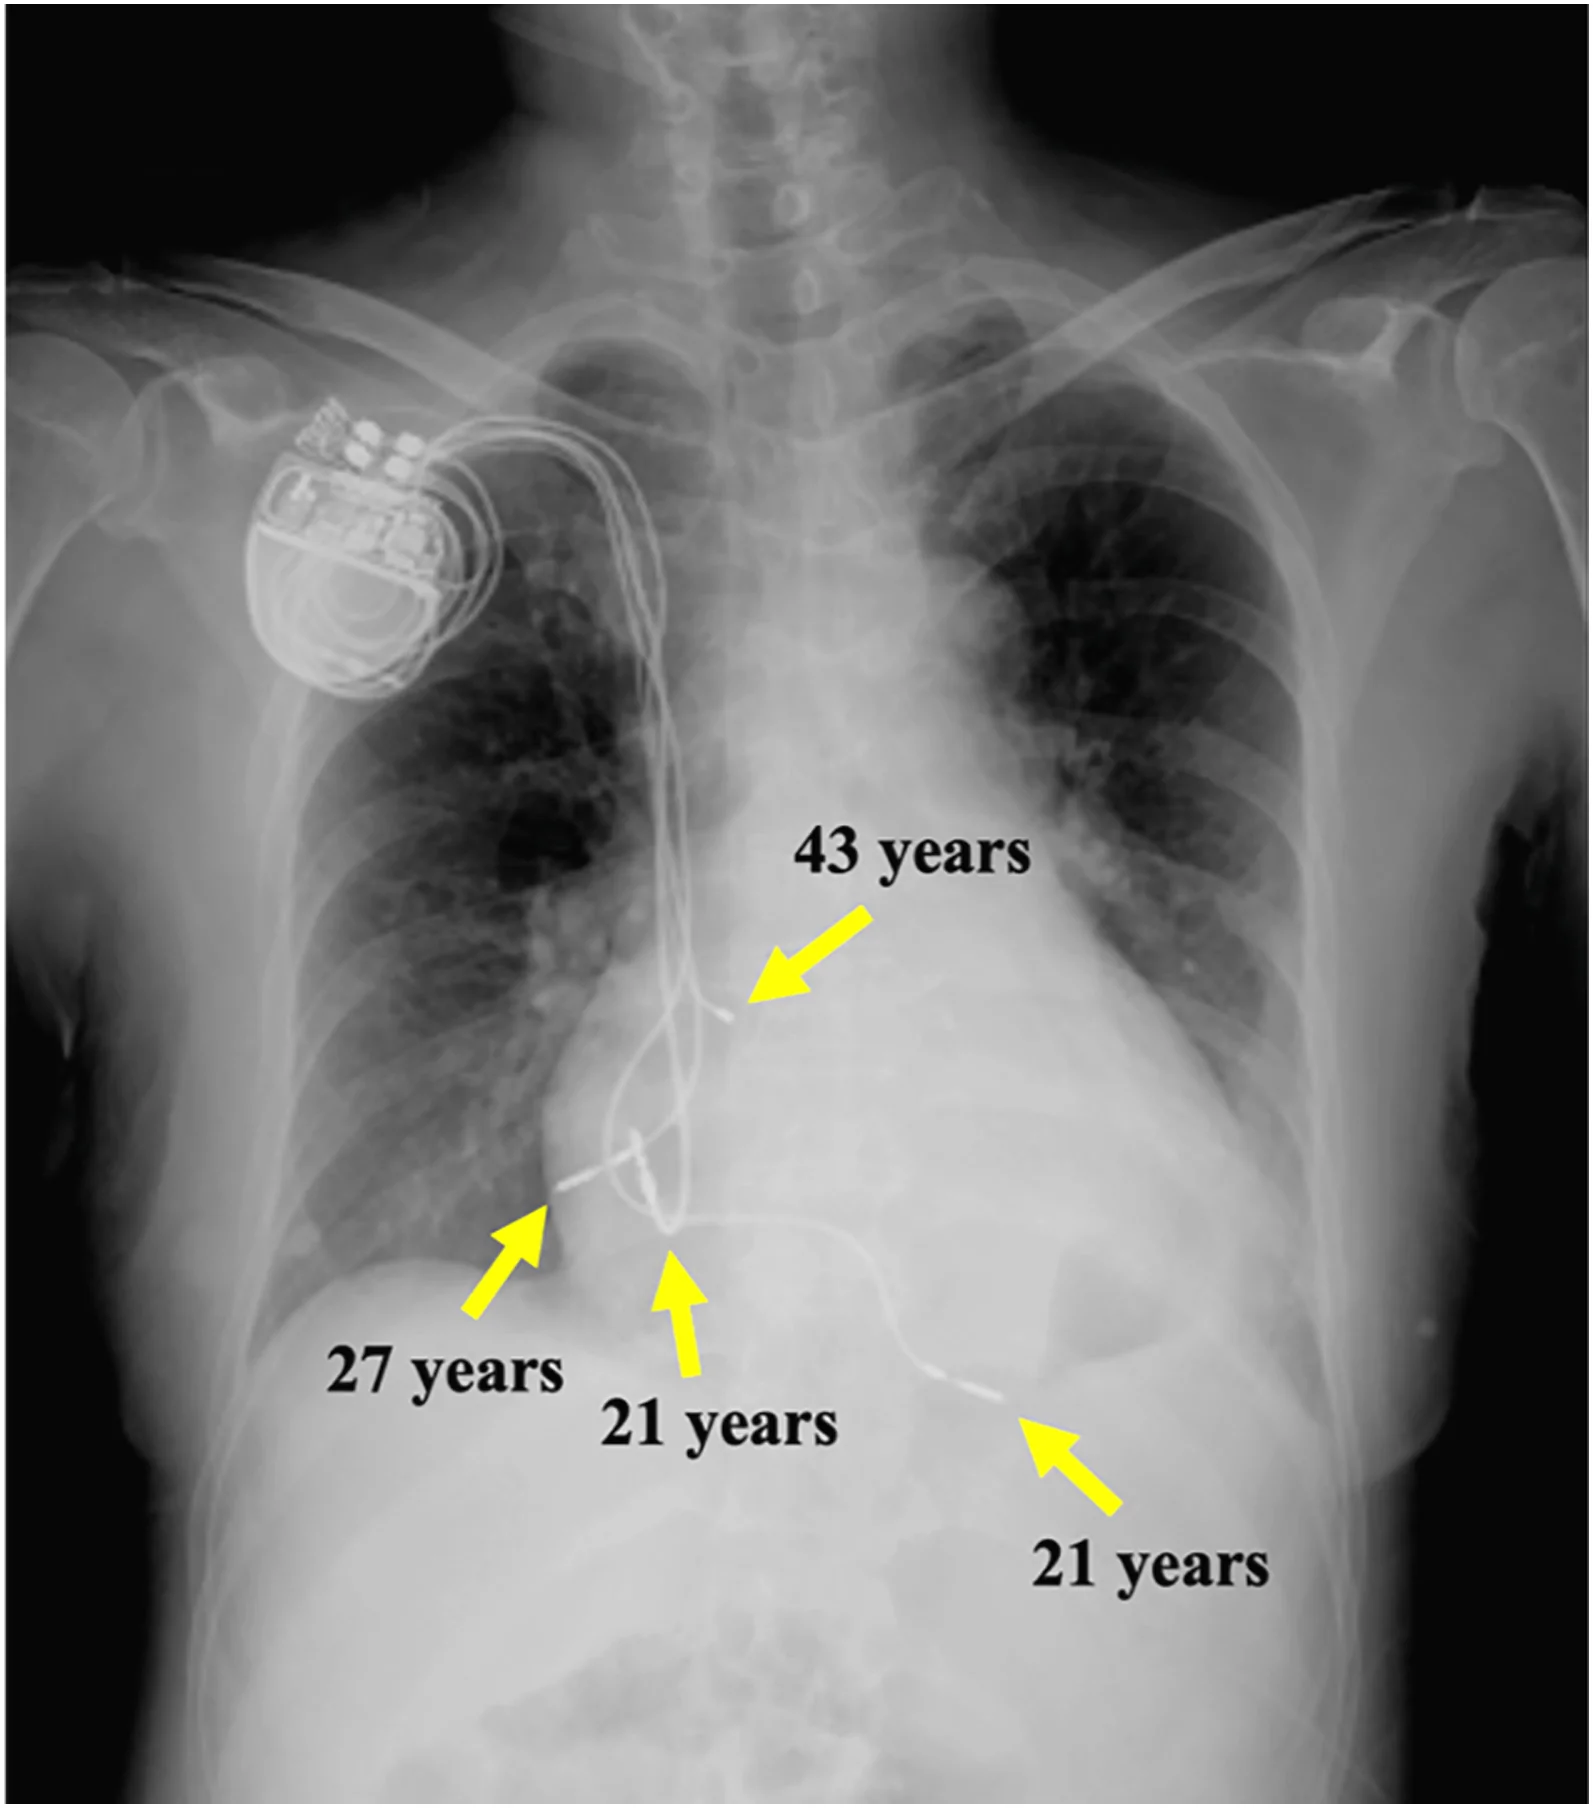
Admission chest radiograph (*anteroposterior seated view*). Right-sided pacemaker system with three leads in the right atrium and one lead in the right ventricle (RV). (*Yellow arrows*) The duration of lead implantation.
